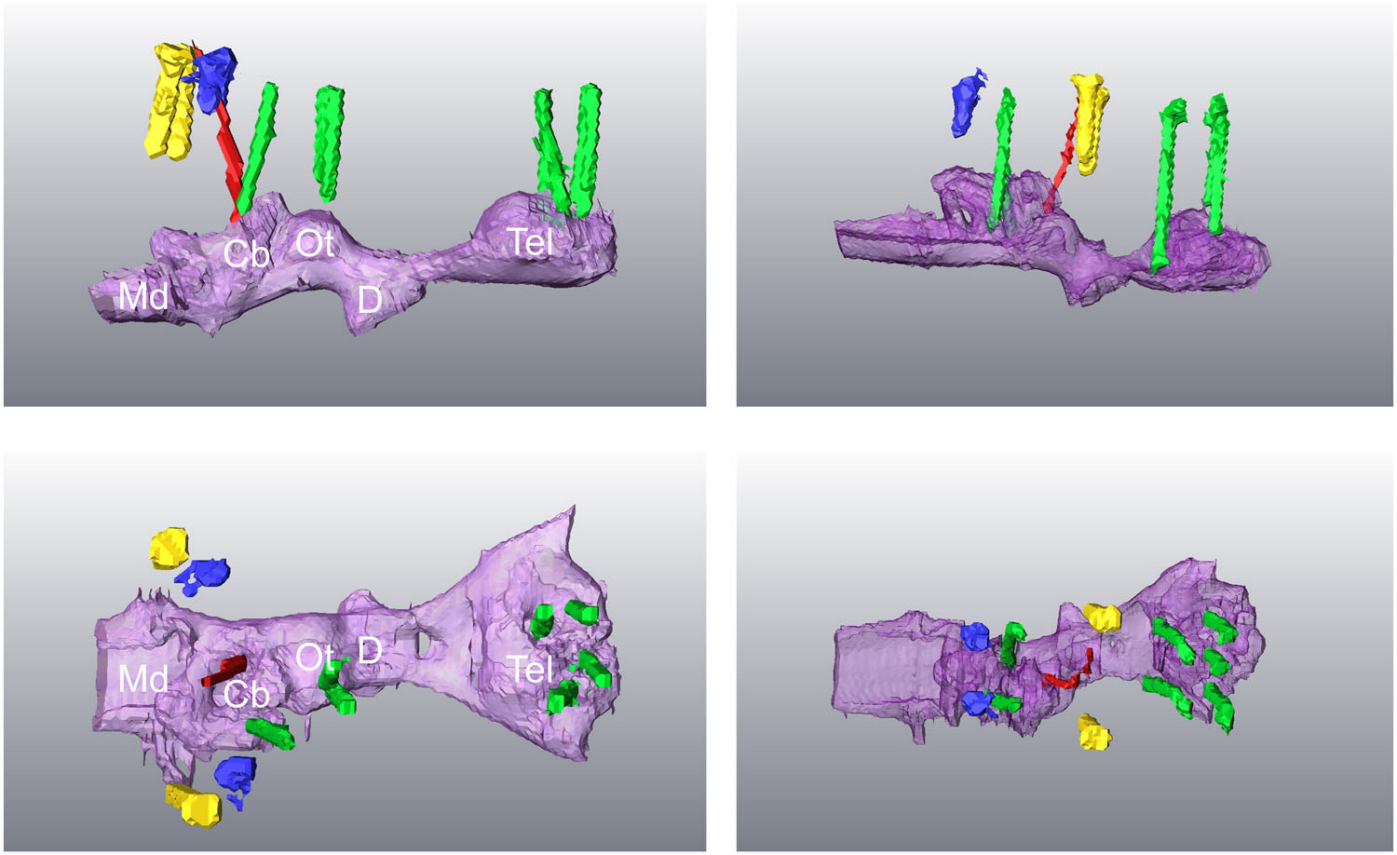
Segmented images from computed tomography (CT) scan for two of the three experimental draughtsboard shark brains from lateral (*top row*) and dorsal (*bottom row*) viewpoints. Imaging of the third shark was not successful. Shown are referential electrodes for the telencephalon, and cerebellum or optic tectum (*green*), reference screws (*blue*), thermistor (*red*), and anchor screws (*yellow*). In all images, the brainstem is on the left. Owing to tissue shrinkage during fixation, it is possible that electrodes seen above the brain here, were touching the brain in vivo. Cb, cerebellum; D, diencephalon; Md, medulla oblongata; Ot, optic tectum; Tel, telencephalon.
